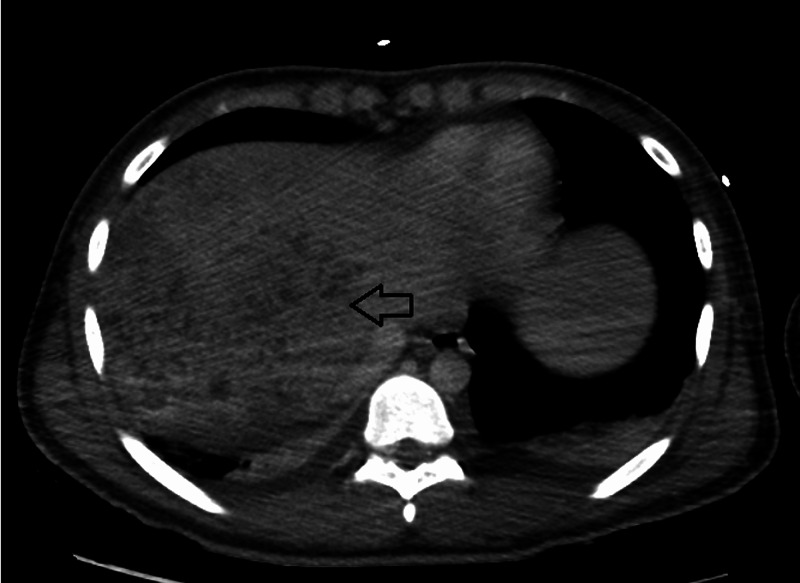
Repeat CT scan showed innumerable poorly defined foci of diminished attenuation in the right lobe of the liver representing multiple hepatic microabscesses.
